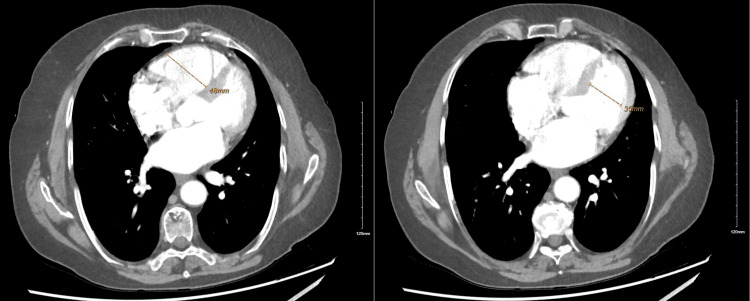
CT pulmonary angiogram. The right ventricle (RV) maximum diameter is 48 mm. The left ventricle (LV) maximum diameter is 35 mm. The ratio of RV to LV is 1.4.
